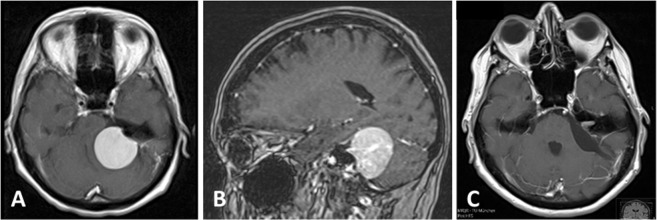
Left-sided *lateral* infratentorial meningioma in a 62-year-old female who presented with hearing loss and had confirmed impaired threshold of the middle frequency range on pure tone audiometry. Preoperative contrast-enhanced T1 MRI in axial (A) and sagittal (B) reconstructions. Postoperative imaging after resection (S2) via a left retrosigmoid craniotomy (C).
